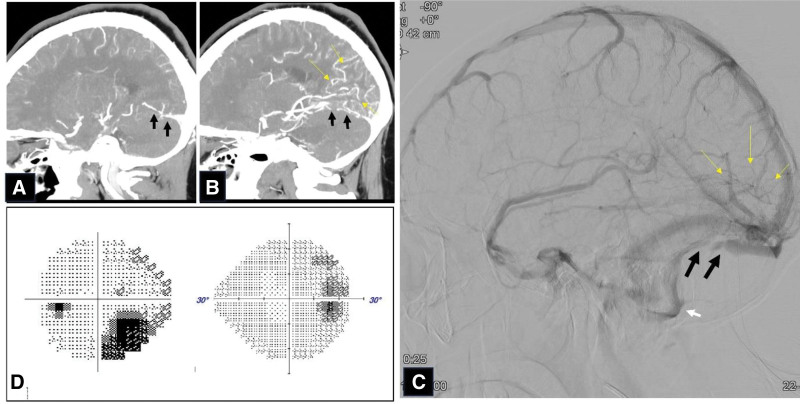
Three-dimensional cerebral CT angiography, 4-vessel angiography following admission, and Humphrey visual field testing following treatment. Three-dimensional cerebral CT angiography reveals contrast well draining through the right transverse sinus (black arrow) (A) and filling defect (black arrow) and superior venous drainage impairment (yellow arrow) (B) on the left transverse sinus. Four-vessel angiography (C) reveals a filling defect of the left transverse sinus (black arrow), indicating the presence of CVT and venous congestion (yellow arrow). However, venous blood flow through the left sigmoid sinus (white arrow) is observed, indicating incomplete occlusion, leading to the decision for medical treatment instead of surgical intervention. Following anticoagulant treatment, the patient’s visual field defect (D) improved significantly compared to the initial right homonymous hemianopia. CT = computed tomography, CVT = cerebral venous thrombosis.
